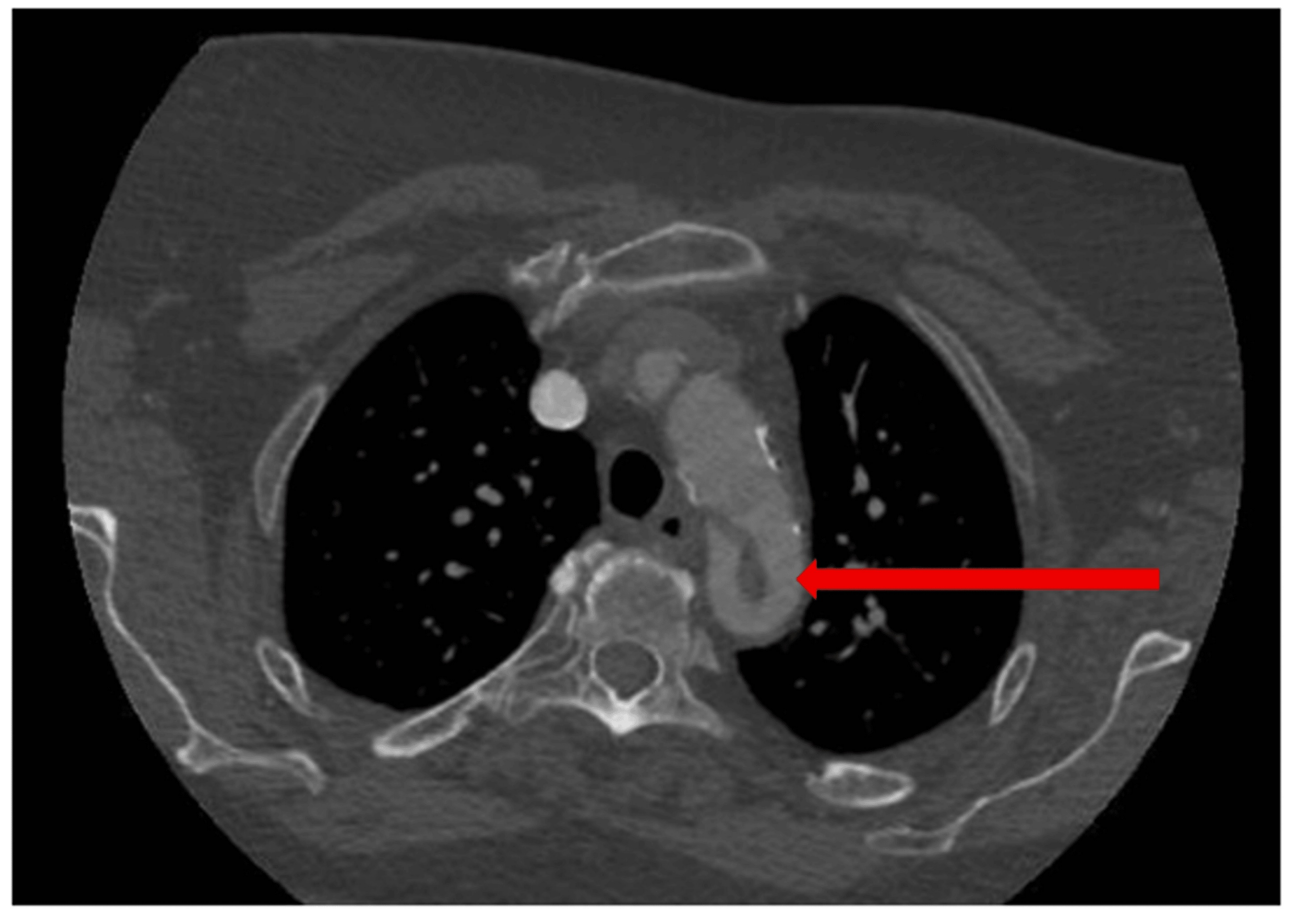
Axial view CTA imaging of the descending thoracic aorta with a mobile thrombus, as indicated by the red arrow, prior to a six-month period of aspirin monotherapy CTA, computed tomography angiography
